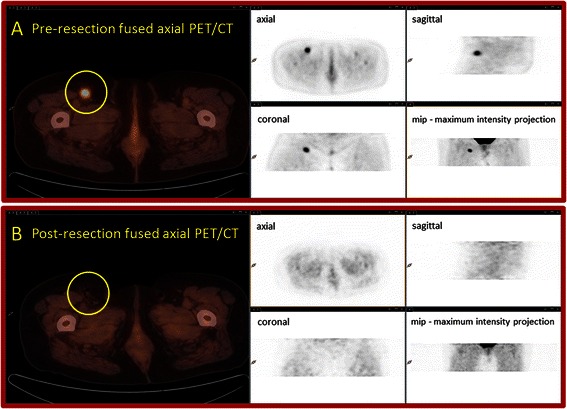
Patient imaging. Panel A: Same-day pre-resection whole body patient PET/CT scan images (i.e., fused PET/CT images and PET images) showing an isolated ^18^F-FDG-avid lymph node (seen within the region of the yellow circle on the fused axial PET/CT image) in the right inguinal region which was not palpable on clinical examination; and Panel B: Same-day post-resection limited field of view patient PET/CT scan images (i.e., fused PET/CT images and PET images) showing successful removal of the intended ^18^F-FDG-avid lymph node in the right inguinal region with residual air in the excision bed (seen within the region of the yellow circle on the fused axial PET/CT image).
